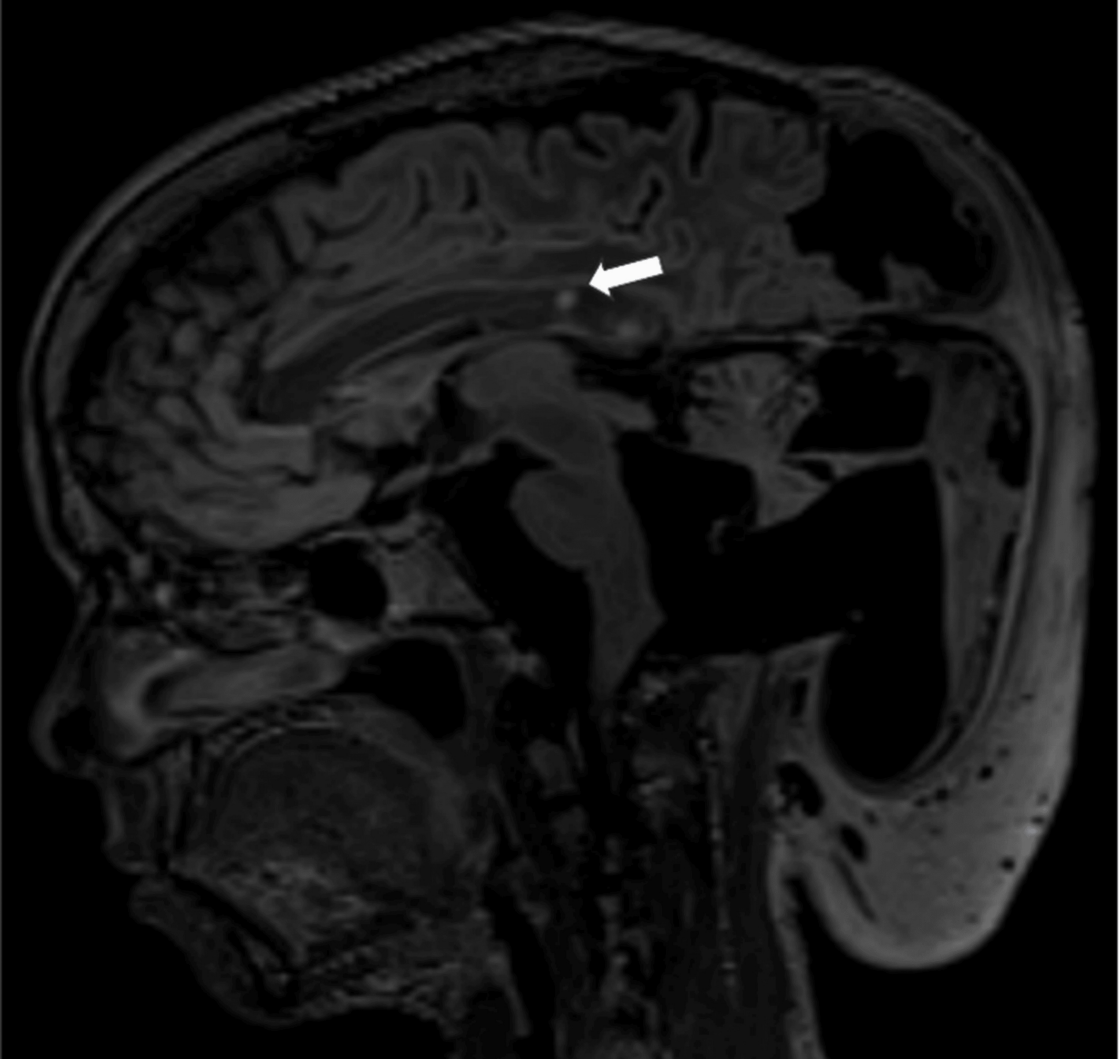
Sagittal fluid-attenuated inversion recovery (FLAIR) images shows focal areas of signal intensities (FASIs) (white solid arrow).
